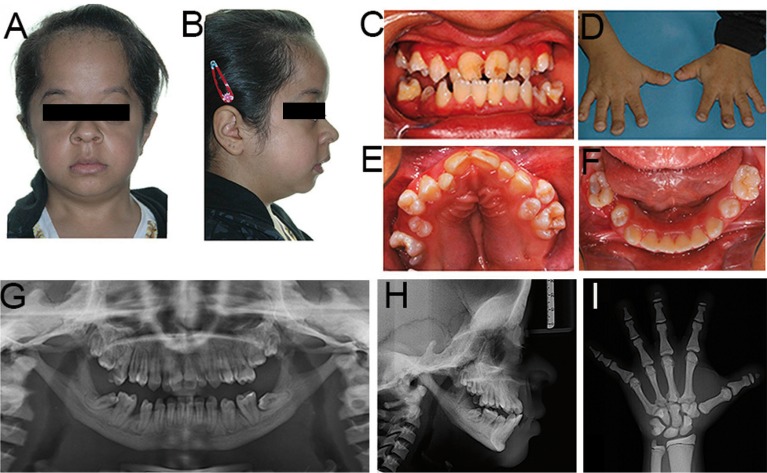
Extraoral (A and B) and intraoral photographs (C, E and F) and photograph of the hands (D). Panoramic radiograph (G), lateral cephalometric radiograph of the head (H), and radiograph of the left hand and wrist (I).
